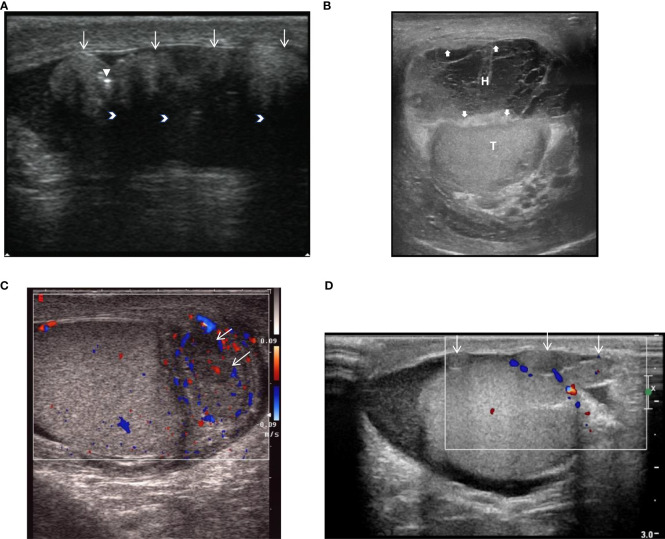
(Ultrasound) (A). Male, 46 years old, Nodular fibrous pseudotumor, Ultrasound shows multiple slightly hyperechoic nodules with involvement of the tunica vaginalis (White slender point),nodules were fused with attenuation behind the nodules (white arrow), and a few punctate calcifications within the lesion (white triangle). (B). Male, 80 years old, diffuse fibrous pseudotumor, ultrasound showing diffuse thickening of the vaginalis wall (white thick arrow), intrathecal thickening separates hydrops (H) and pushing testis (T). (C). Male, 50 years old, nodular fibrous pseudotumor of tunica tunica testis in cauda epididymidis with epididymitis. Ultrasound showed that the cauda epididymidis was enlarged, with 2 hypoechoic nodules (white slender arrows) in size, and rich blood flow signals in the epididymis. (D). Case8, male, 40 years old, nodular fibrous pseudotumor, ultrasound clearly showed multiple isoechoic nodules (white slender arrows) in the wall of the sheath without abundant blood flow signals.
